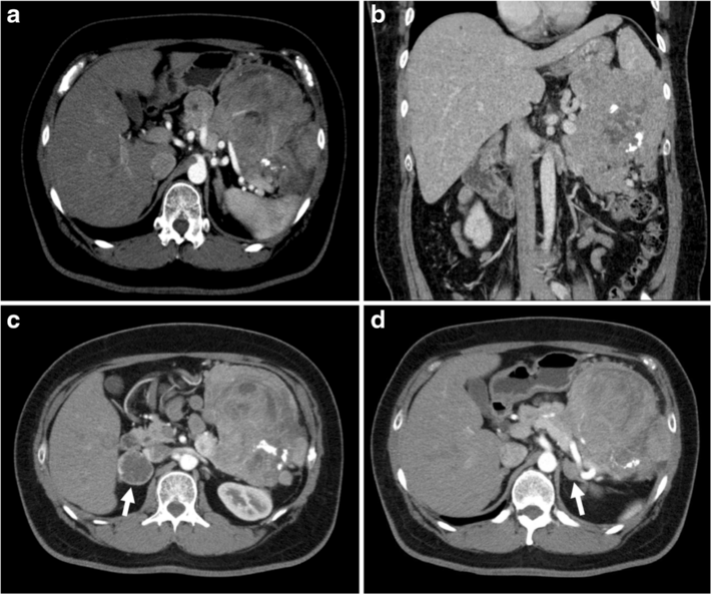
a, b Computed tomographic image of the abdomen, demonstrating a large was heterogeneously enhanced insulinoma arising from the tail of the pancreas. c Computed tomographic image of the abdomen, demonstrating a ring-enhanced tumor of the right adrenal gland (*arrow*). d Computed tomographic image of the abdomen, demonstrating a poor-enhanced tumor of the left adrenal gland (*arrow*)
